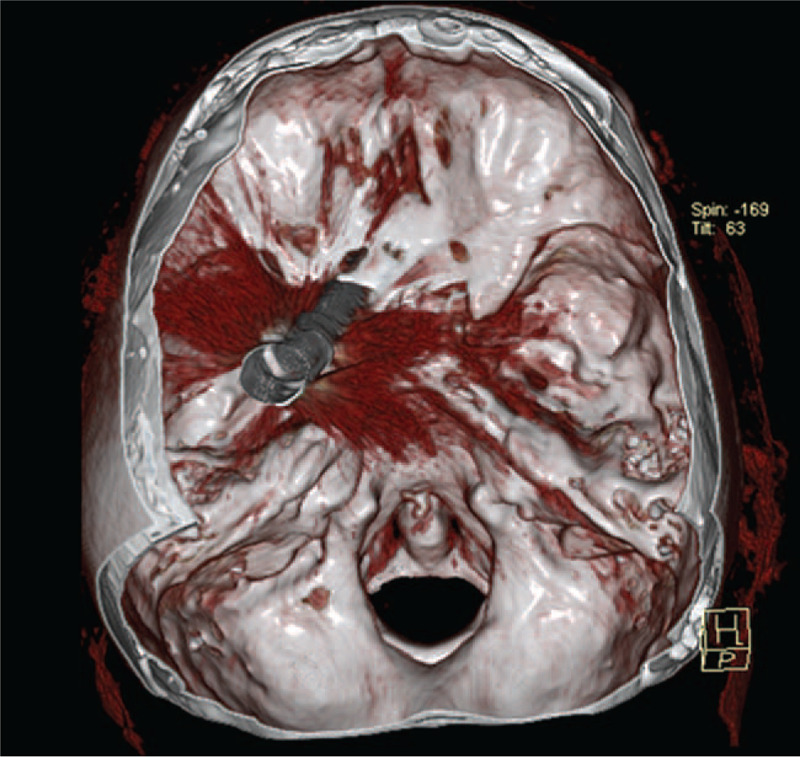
CT images. The metallic object penetrated the cranium and crossed the entire left cerebral hemisphere. The tip of the harpoon was embedded in the left upper parietal cranium, with a displaced fracture and a slight exocranial protrusion of the stumps. (2A Coronal CT scan; 2B Sagittal CT scan).
